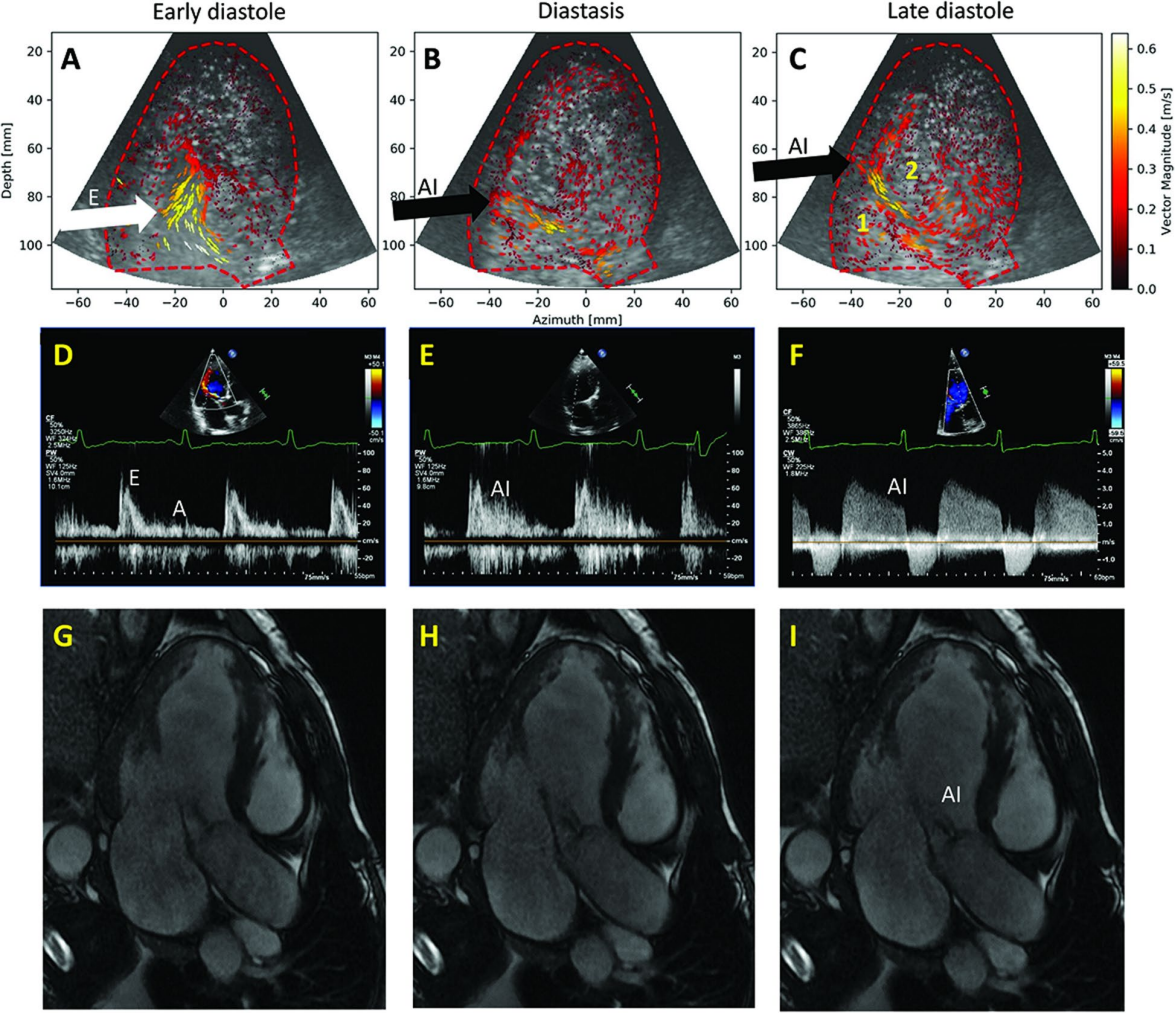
Patient 4. A-C: HFR echoPIV shows an AI jet having a perpendicular course to the ultrasound beam, making it harder to assess with colour Doppler. A local counter-clockwise vortex formed under the mitral valve (C:1) and another clockwise vortex formed in the mid-LV (C:2), leading to recirculation of blood from the AI and mitral inflow high-velocity AI jet limiting the mitral inflow (white arrow); D-E: PW mitral inflow, interference with the AI; F: AI jet on CW Doppler; G-I: CMR, confirming the AI jet pushing the anterior mitral valve
